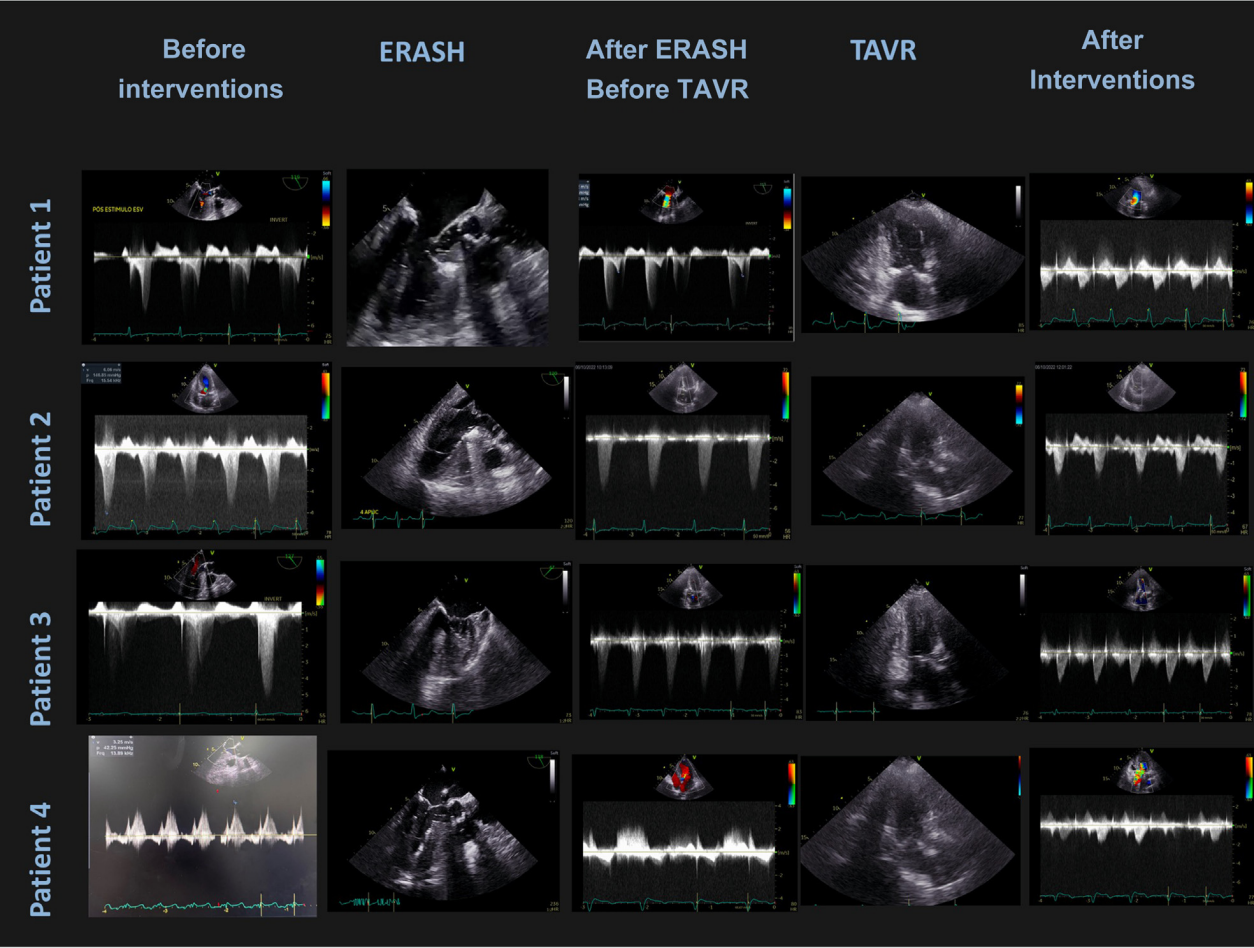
Complete Echocardiographic Images of the 4 Cases in Each Step of Treatment Images were acquired for each patient in the different steps of the treatment. The images in the first column present echocardiography before interventions, with 2 distinct peaks of obstruction: one earlier representing valve obstruction and one later “dagger-shaped” representing LVOT obstruction. The second column shows TEE images taken during septal ablation. It is possible to visualize the catheter inside the LVOT. The third column shows the absence of LVOT gradient before TAVR, but significant aortic valve gradient remains. The fourth columns shows an intraoperatory TAVR TTE image. The last column shows the gradients after all interventions were successfully performed. LVOT = left ventricular outflow tract; TAVR = transcatheter aortic valve replacement; TEE = transesophageal echocardiogram; TTE = transthoracic echocardiography.
